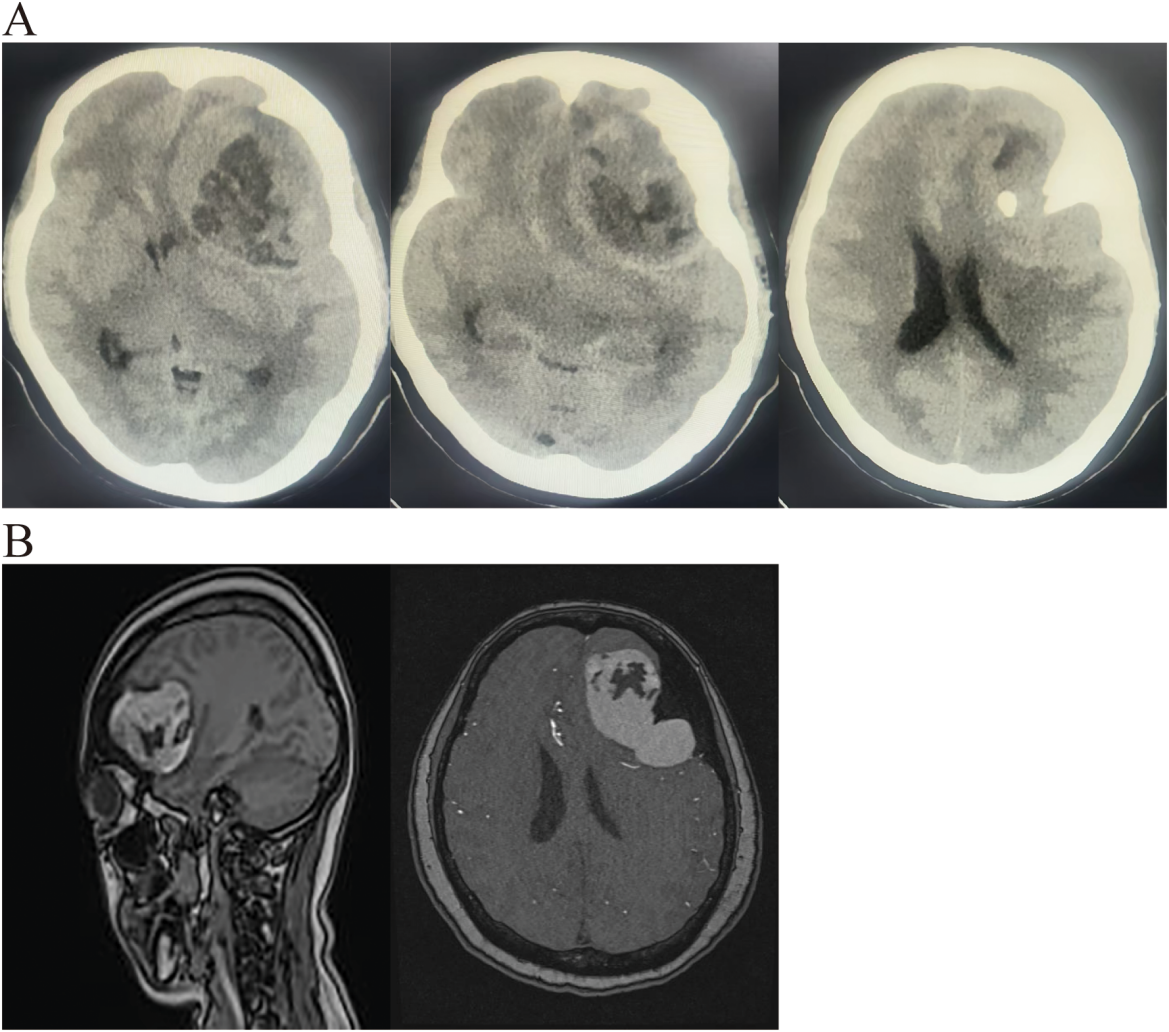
(A) Pre-operative CT revealed a lobulated, mixed-density lesion in the left frontotemporal lobe with associated hyperostosis of the left frontal bone. (B) Pre-operative MRI demonstrated a lobulated, mixed-intensity lesion in the left frontotemporal lobe consistent with an epidermoid cyst.
